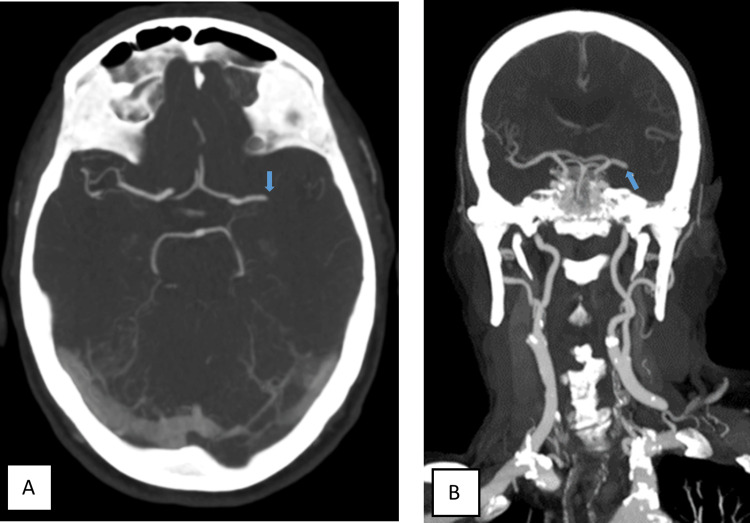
Axial (A) and coronal (B) CT angiography showing the presence of an endoluminal thrombus in the M1 segment of the left middle cerebral artery (blue arrow) CT: computed tomography
